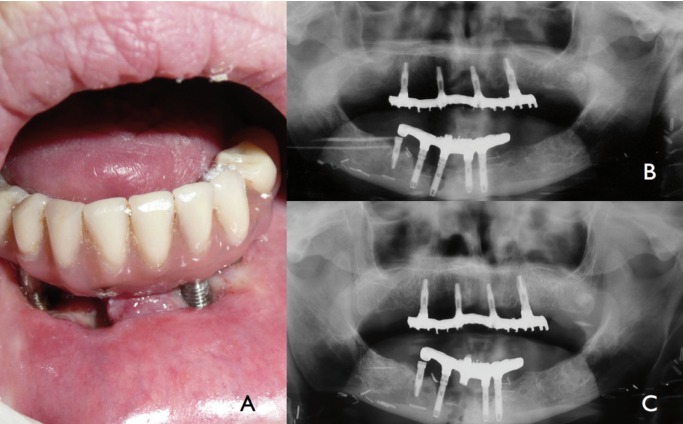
Case 1: A) Intraoral view of clinical periimplant disease implant body exposure. B) Advanced periimplant disease in orthopantomography around three mandibular implants. Maxillary fixed prothesis supported by four implants without signs of PI. C) Central implant removed and prothesis fixed again.
